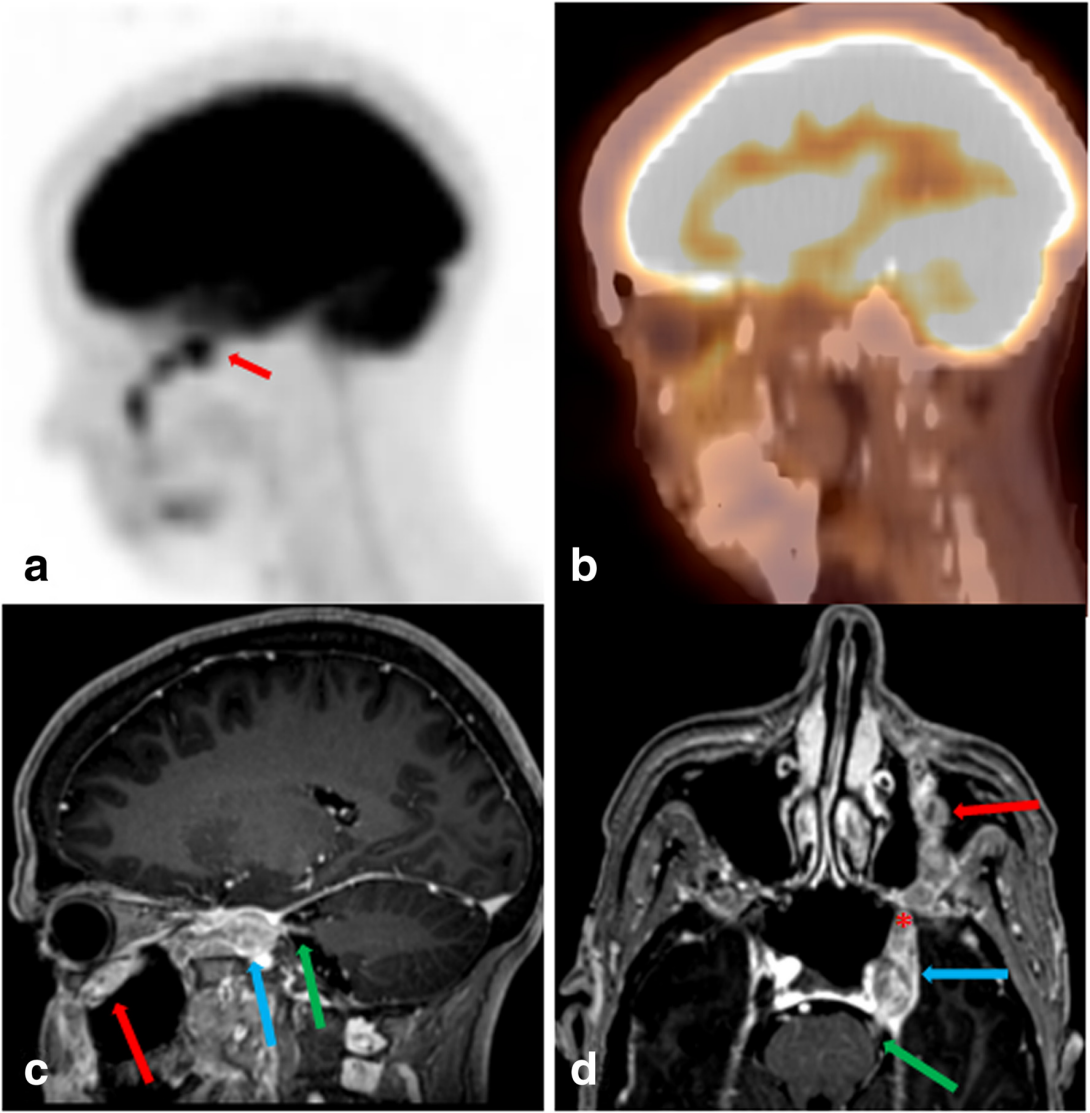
A 50-year-old female who is status post excision of left nasolabial melanoma,presenting with left perioral numbness. Sagittal unfused (a) and fused (b) FDG PET/CT shows alongitudinal hypermetabolic mass extending retrogradely along the expected course of theinfraorbital branch Of V2 in the orbital floor, with intracranial extension (arrow). Sagittal (c), axial (d) curved Multiplanar reformatted images (MPR) of fat suppressed post contrast T1 WI MRI confirming retrograde perineural metastatic melanoma, along the infraorbital branch of V2 (red arrow), through the foramen rotundum (asterix), into the cavernous sinus (blue arrow), to the cisternal portion of the left trigeminal nerve (green arrow)
